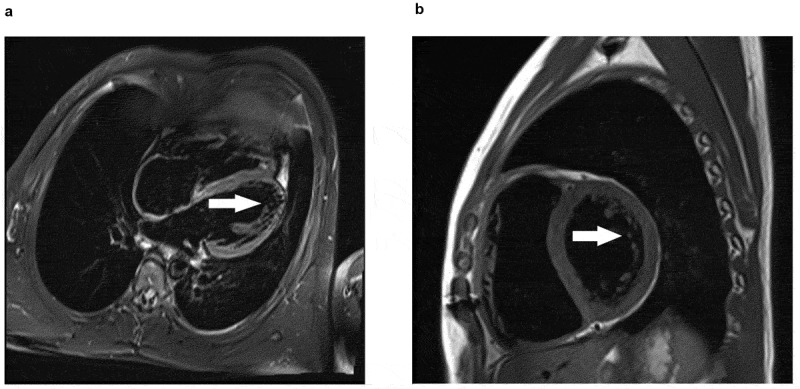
Cardiac magnetic resonance of the patient with LVNC. Fat-suppressed T2-weighted imaging shows a non-compacted myocardium.
